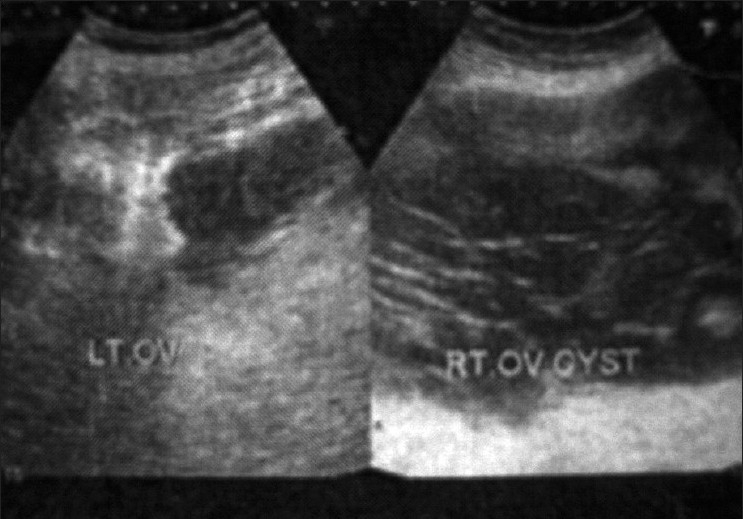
Ultrasonography of the abdomen (pre-operative) showing cystic lesion with internal septation measuring 155 mm×175 mm in the right ovary and a small cyst was also seen in the left ovary
